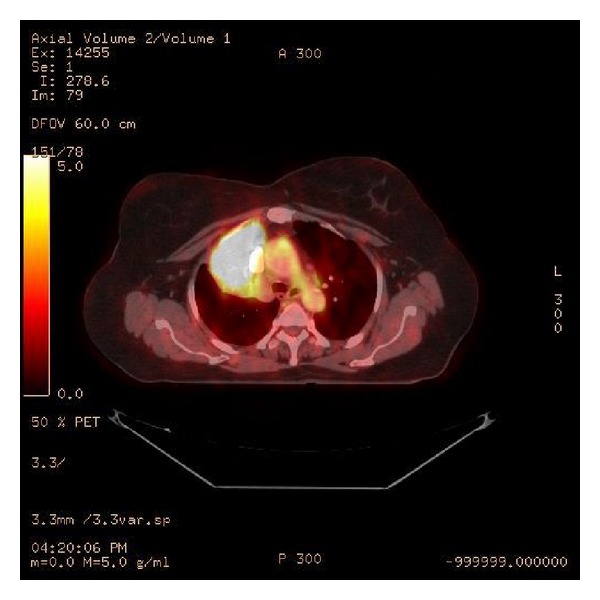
PET SCAN image showing FDG avid large right upper lobe heterogeneous low-density mass causing mass effect upon the main stem bronchus.
